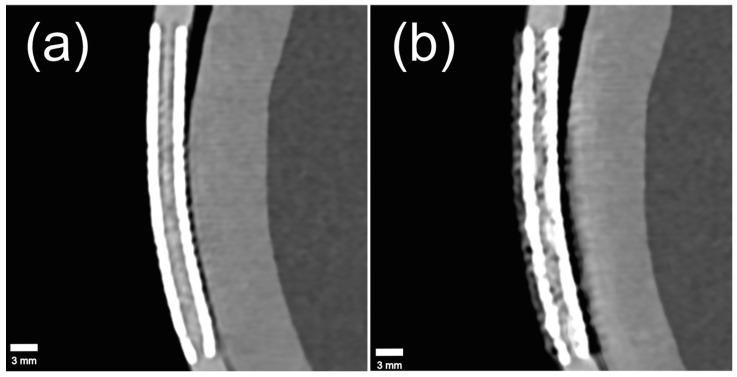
Longitudinal slice of a stent (Boston Scientific Promus Element (Boston Scientific Corporation, Marlborough, MA, USA)) at rest (a) and in motion (b). PCD CT in SR mode using the Bv60 kernel. The window width was set to 1500 HU and the center to 300 HU. The longitudinal slice is just for demonstration purposes to better show the motion artifacts. The measurements were made in the axial slices.
